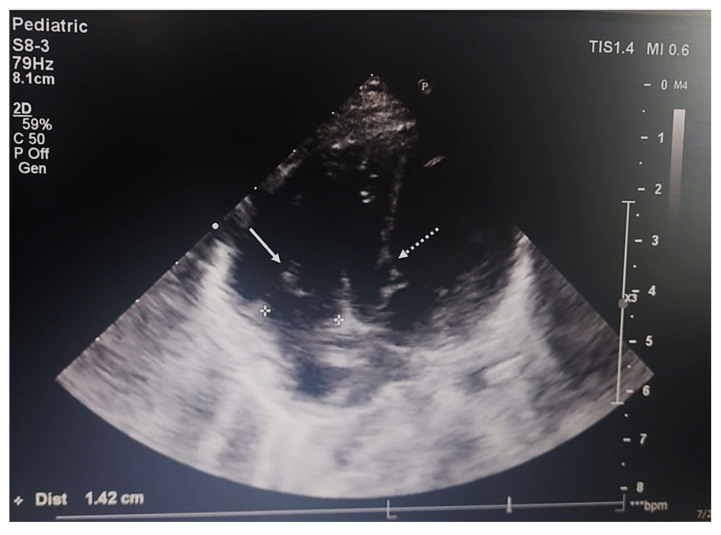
Preoperative transthoracic echocardiography, four-chamber view; the continuous arrow indicates a mass located on the tricuspid valve, which was diagnosed as an arteriovenous hemangioma; the dotted arrow shows a ventricular septal defect that was resolved surgically.
